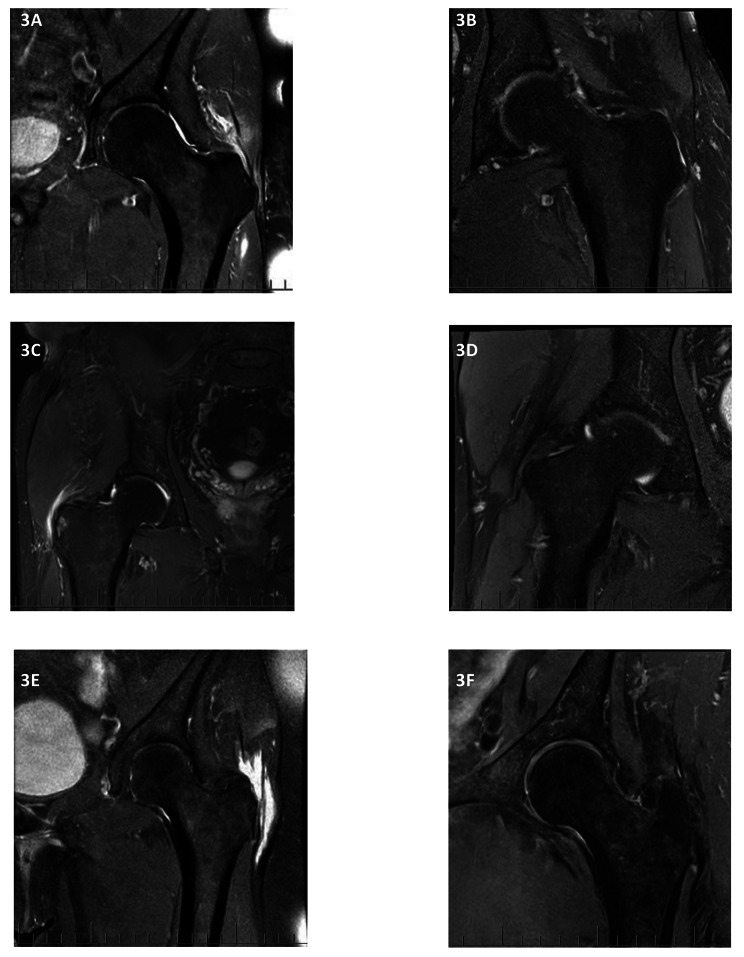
Pre- and post- MRI images from three participants who had improvement of more than 2 points in their MHIP scores. 3A and 3B are the pre- and post-treatment scans for participant 64; 3C and D for participant 70, and 3E and 3F for participant 33 respectively
